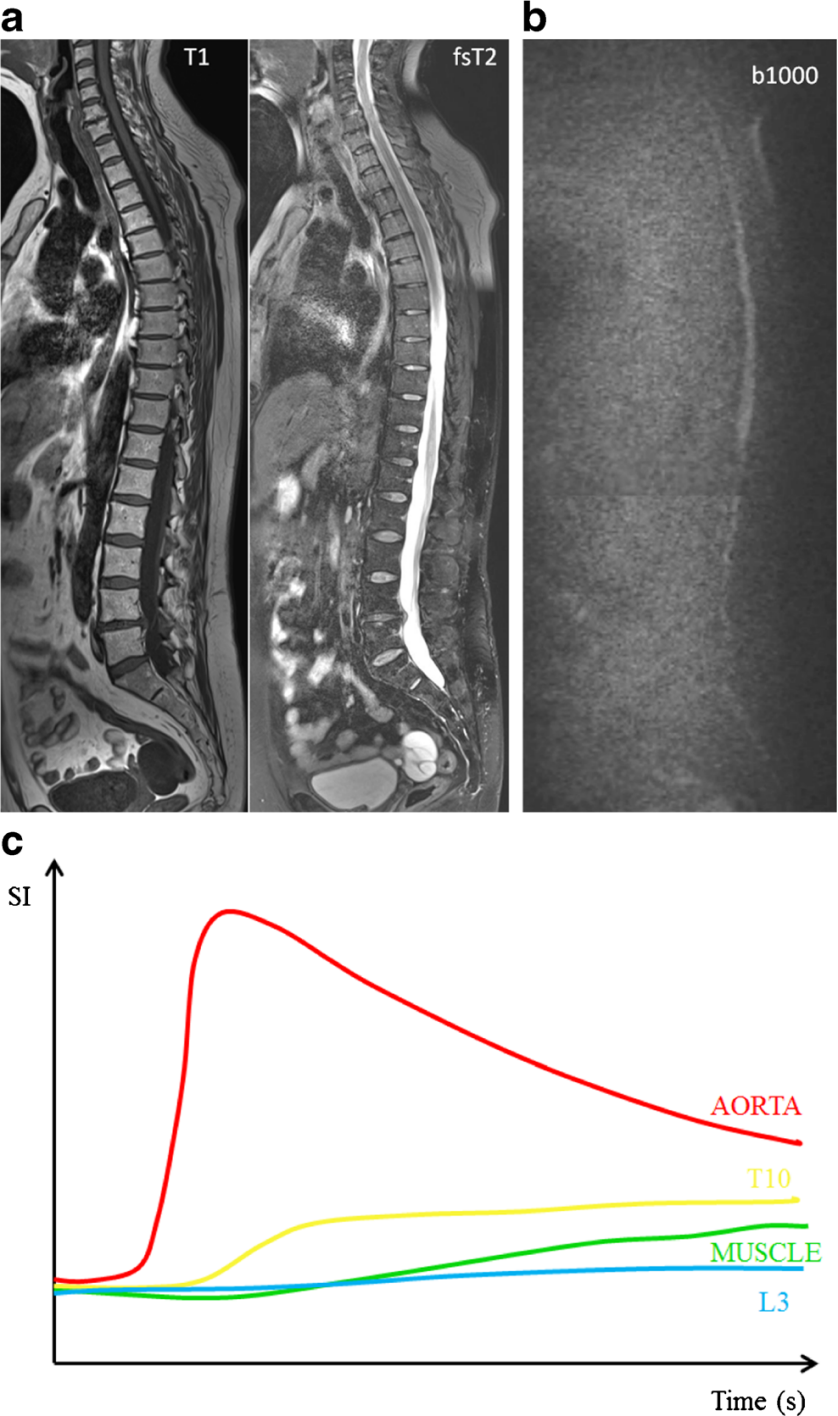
MR images of a patient with smouldering multiple myeloma, 15 % plasma cells on bone marrow biopsy. a T1-weighted and fat-suppressed T2-weighted images of the thoracolumbar spine displaying altered bone marrow signal intensities, with slight diffuse signal decrease on T1 images without significant changes on T2-weighted images. b Normal signal intensity on b1000 images, due to the residual high amount of fat cells in the bone marrow. c Time-intensity curve derived from DCE-MR imaging with TIC type 1, corresponding to a normal vascularization of the bone marrow without signs of neoangiogenesis
